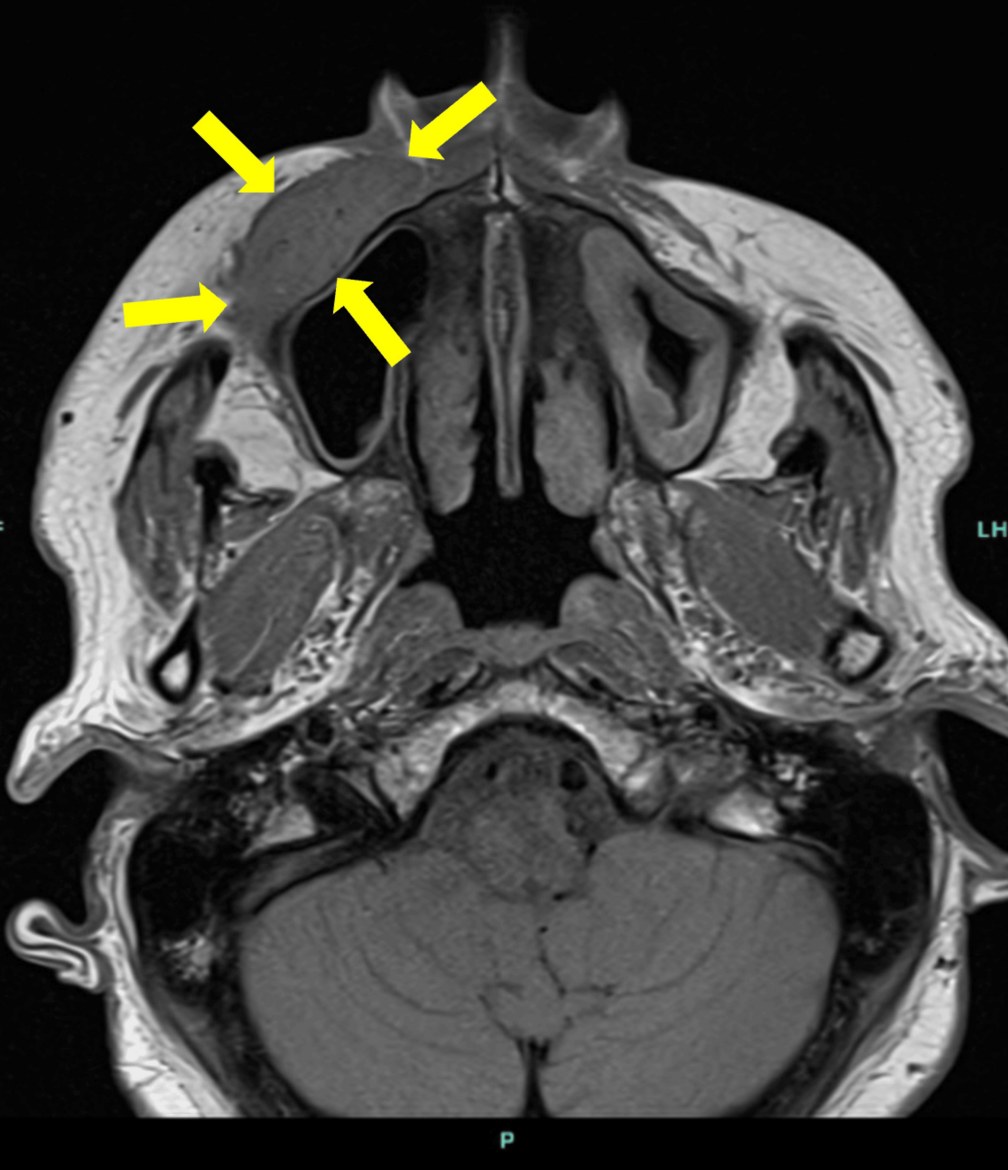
Coronal T1-weighted magnetic resonance imaging of the right facial mass (arrows) prior to surgical treatment. There is a well-delineated soft tissue mass within the right cheek soft tissue measuring approximately 3.5 x 1.5 cm in maximal length and depth.
